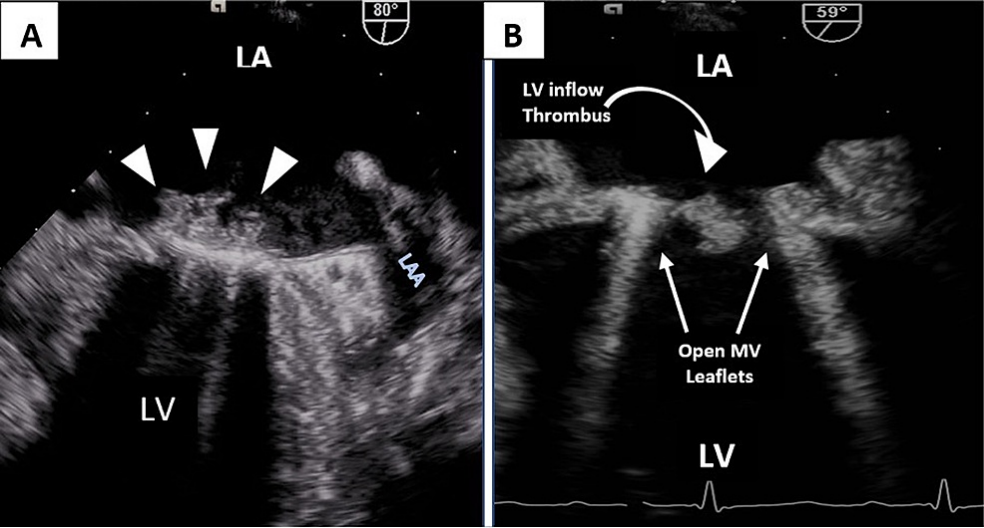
Transesophageal echocardiogram Initial transesophageal echocardiogram showing (A) multiple sessile thrombi (arrowheads) on mitral valve annulus and (B) diastolic protrusion of a large mobile thrombus (curved arrow) into LV inflow between open prosthetic MV leaflets. LA = left atrium, LAA = LA appendage, LV = Left ventricle, MV = mitral valve
